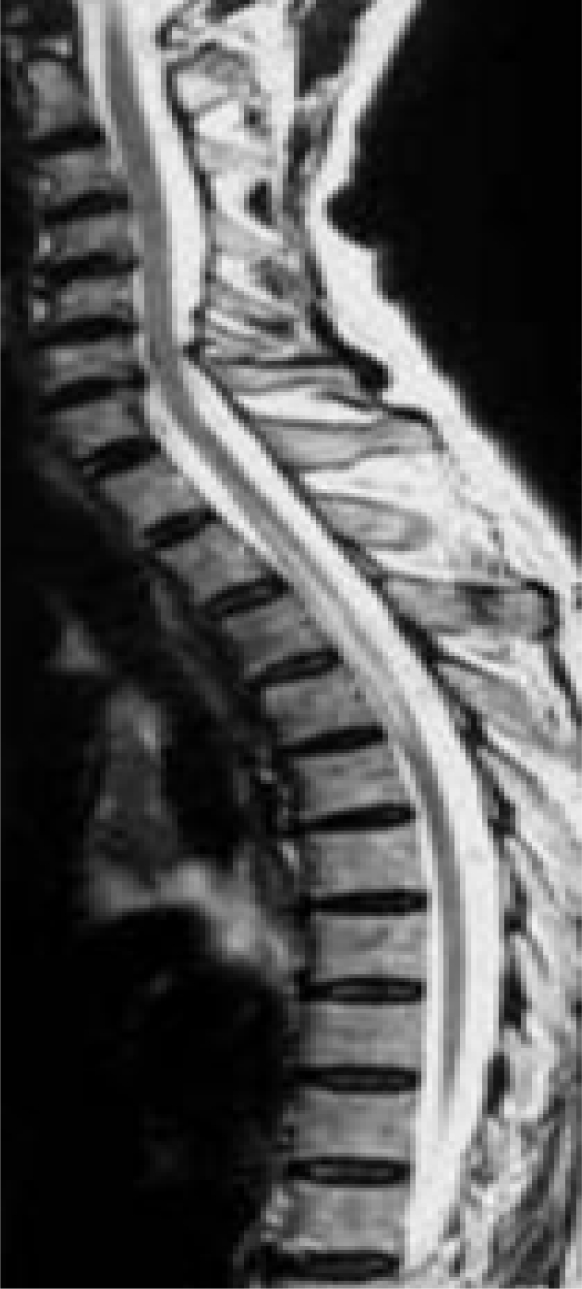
T2 Sagittal spine MRI of a 30 year old lady presented to our hospital with left lower extremity weakness and low back pain showing the typical fusiform cord signal in TM.
